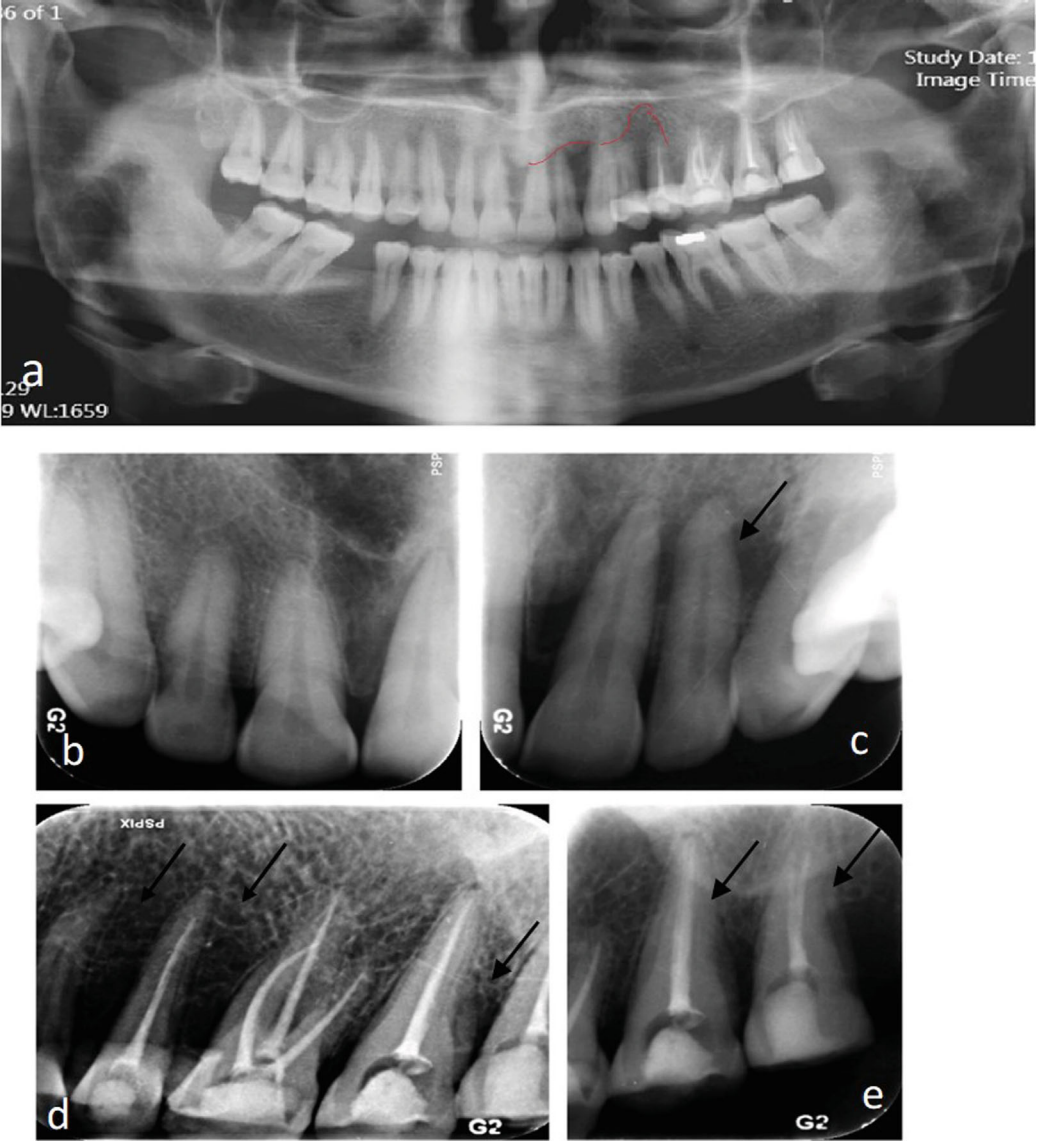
(a) Panoramic radiograph showing bone destruction in the right maxillary quadrant. (b) Periapical view of the right maxillary anterior teeth. (c) Periapical view of the left maxillary anterior teeth: the arrows show the bone resorption around Teeth 21 and 22. (d, e) Periapical radiographs of the left maxillary region: the arrows show the severe bone destruction around the root-filled maxillary premolars to the third molar.
